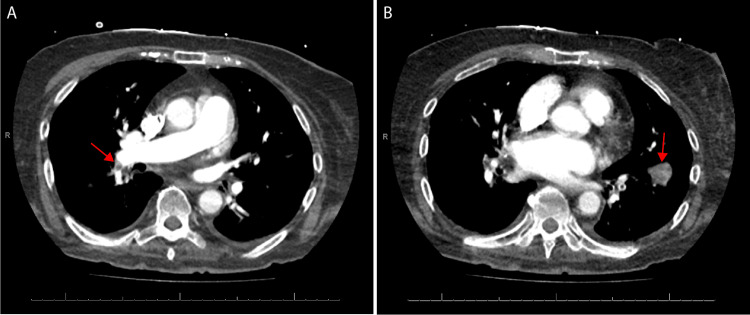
Computed tomography (CT) pulmonary angiogram depicting (A) emboli in the right main pulmonary artery, extending into the right lower lobe and (B) large emboli extending into the left lower lobe.
